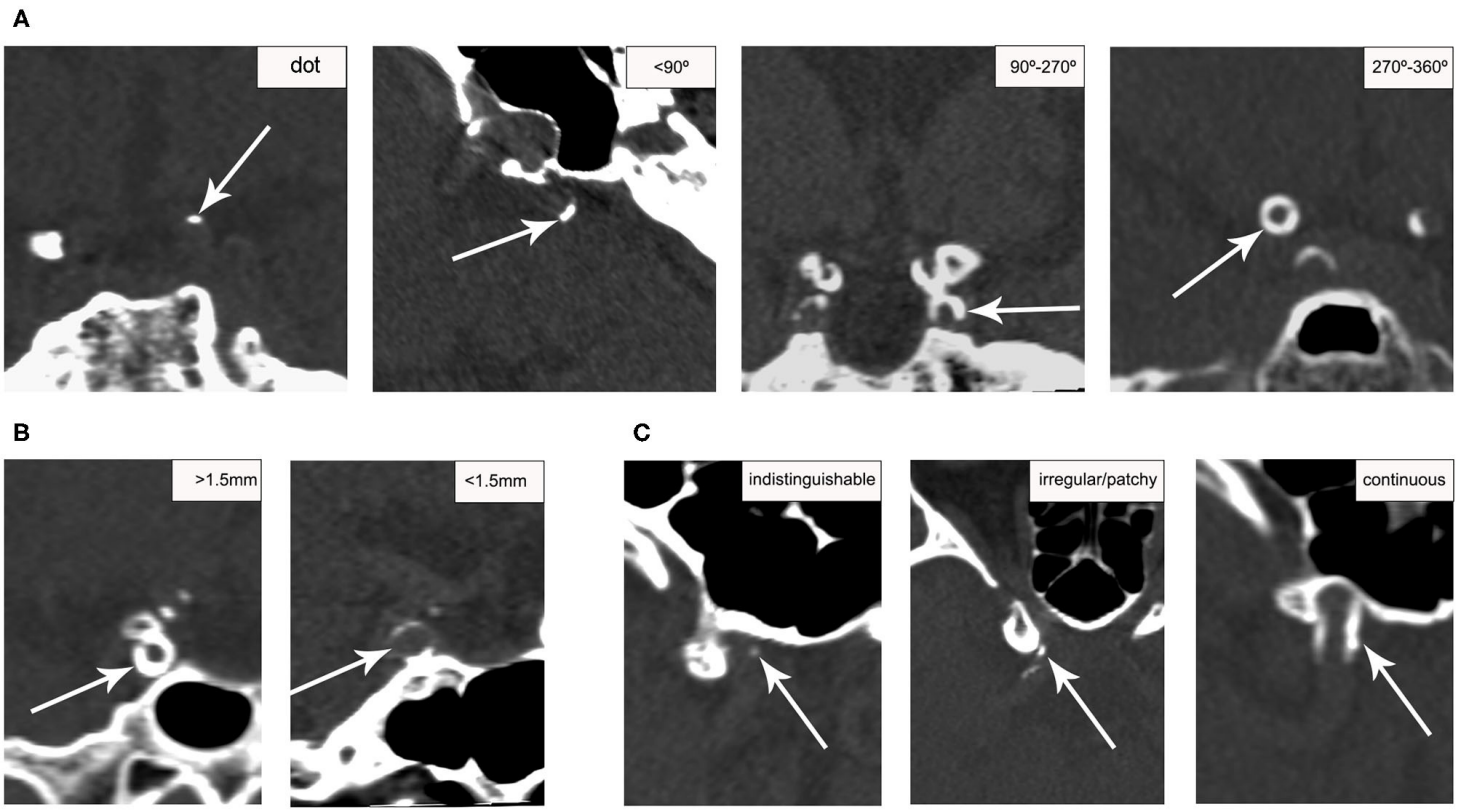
Examples of calcification score for categorizing ICA calcification patterns. Calcification (arrows) was scored based on circularity (A), thickness (B), and continuity (C) on CT. Circularity and thickness were assessed on the short axis; continuity was assessed on the long axis.
